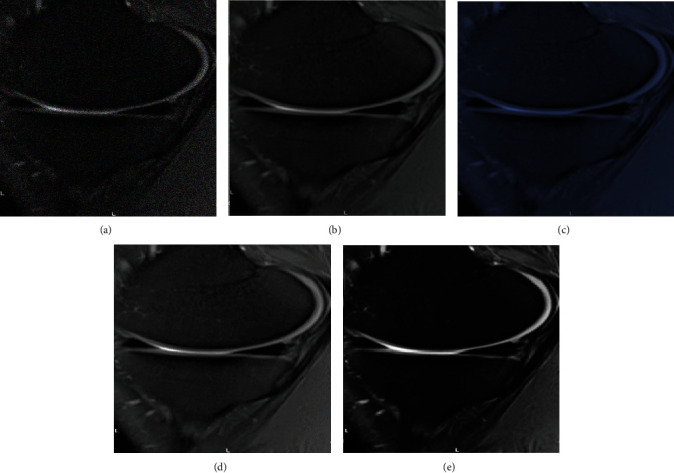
Reconstruction results of MRI images by different algorithms. (a)–(e) are the original image, reconstructed image by SRCNN algorithm, reconstructed image by SSD algorithm, reconstructed image by EDSR algorithm, and reconstructed image by the model in the study.
